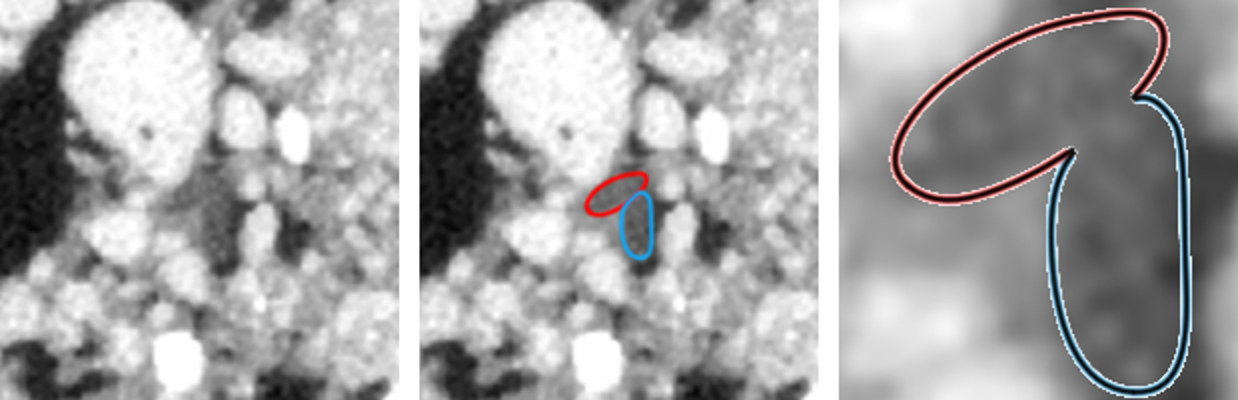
Two interacting roots. Two interacting roots originating from different root systems: the boundary between them is unidentifiable: (a) microcomputed tomography image; (b) root sections marked; (c) zoom in to the root objects.
